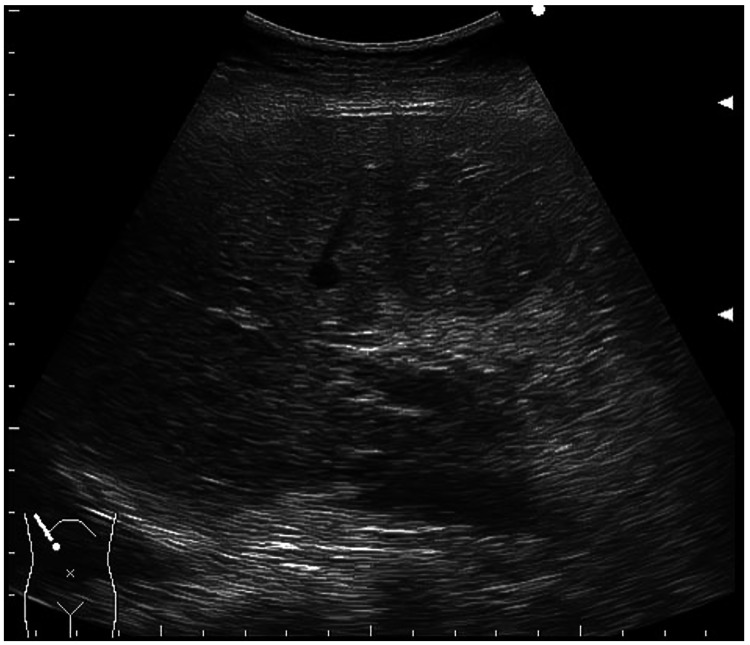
Resolution of portal vein gas after surgery Follow-up abdominal ultrasound performed within 24 hours after resection of necrotic ileum showed the complete disappearance of portal vein gas within the liver parenchyma.
